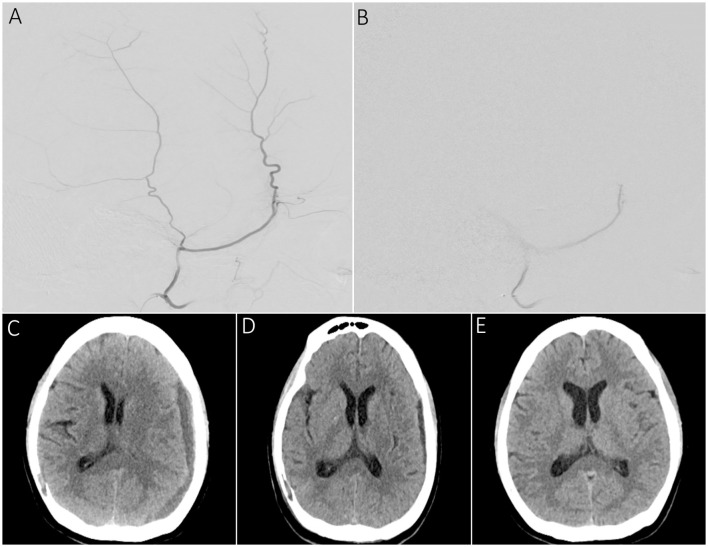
A sample patient presenting with dysarthria and right-hand tingling undergoing MMA embolization for chronic SDH. (A) Supra-selective lateral angiography showing the frontal and parietal branches of the MMA before the embolization procedure. (B) Supra-selective MMA angiography demonstrating persistent proximal main trunk occlusion following PVA particle embolization. (C) Pre-embolization CT scan showing a left convex cSDH with a maximum width of 12 mm and a midline shift of 5 mm. (D) 2-week follow-up scan showing a reduction in maximum width of the SDH up to 6 mm and resolution of prior midline shift. (E) 3-month follow-up scan demonstrating complete resolution of cSDH.
